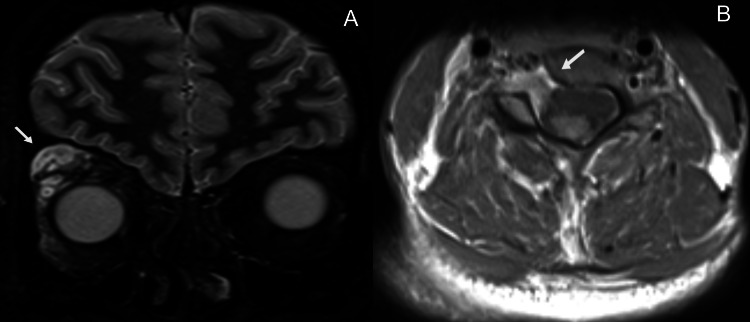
Brain (A) and cervical cord (B) magnetic resonance imaging. (A) On a coronal short tau inversion recovery (STIR) sequence, a globally hyperintense lobulated mass can be seen in the extraconal compartment of the right orbit, in keeping with a plexiform neurofibroma (arrow). (B) On an axial view of a T1-weighted turbo spin echo sequence, after administration of gadolinium, an enhancing mass in the right foramen of a cervical vertebrae can be depicted, suggesting a peripheral neurofibroma (arrow).
